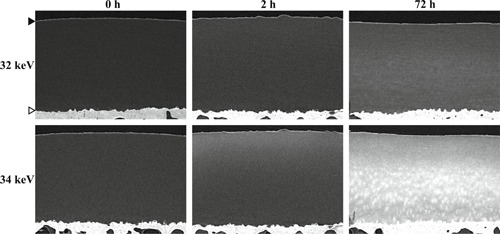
Tomographic slices of the computed tomography (CT)‐reconstructed volume acquired with 32 and 34 keV photon energies at different time points (0, 2, and 72 h) after the contrast agent immersion. The articulating surface and cartilage–bone interface are marked with black and white arrowheads, respectively. The contrast agent mainly responsible for inducing the contrast is CA4+ and gadoteridol for 34 and 32 keV images, respectively
